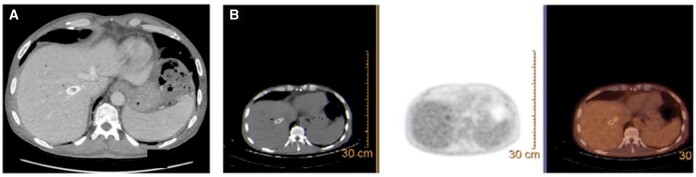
(A) Contrast enhanced CT scan, venous phase, showing thrombotic material within the intra-parenchymal portion of the endoprosthesis; (B) PET with 2-deoxy-2-[fluorine-18]fluoro-D-glucose showing FDG avid uptake within the thrombosed TIPS.
